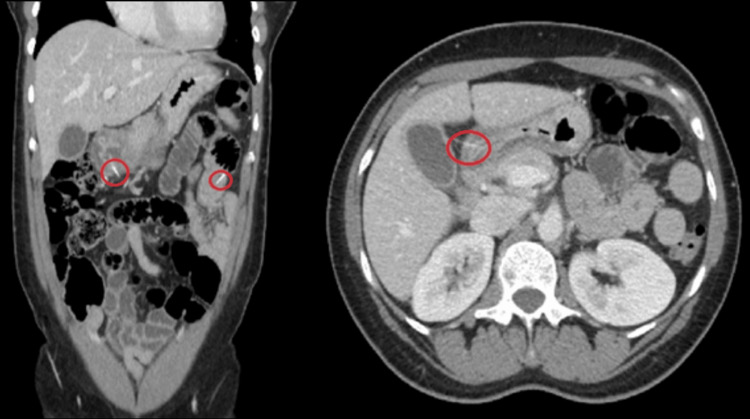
Initial CT scan showing gastric and jejunal foreign bodies
